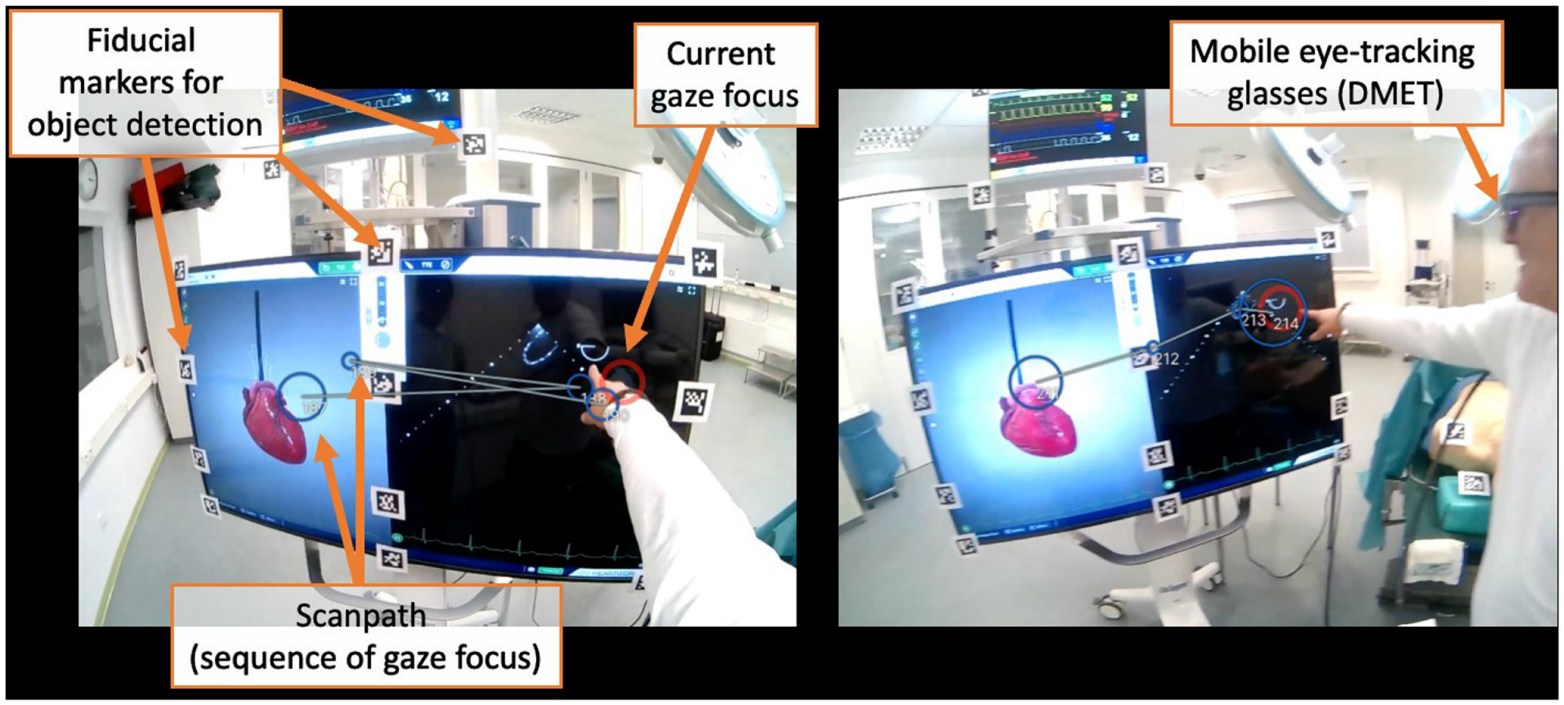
Example of a DMET setup during ultrasound training in an operating room. In this scenario, both the teacher and the student are wearing mobile eye-tracking glasses to track their eye movements. The image showcases the respective gaze focus of both the teacher (left) and the student (right) from their individual perspectives (red circle). At this particular moment, both are observing the ultrasound image displayed on the right side of the monitor, initiated by the teacher via finger pointing. The scanpath (the sequence of gaze focus) can be visualized afterward, which allows for detailed analysis of gaze patterns over time. The QR codes visible in the scene serve a crucial purpose in automated object detection. They enable the system to distinguish between different areas of interest, such as whether the gaze is focused on the anatomical image or the ultrasound image.
